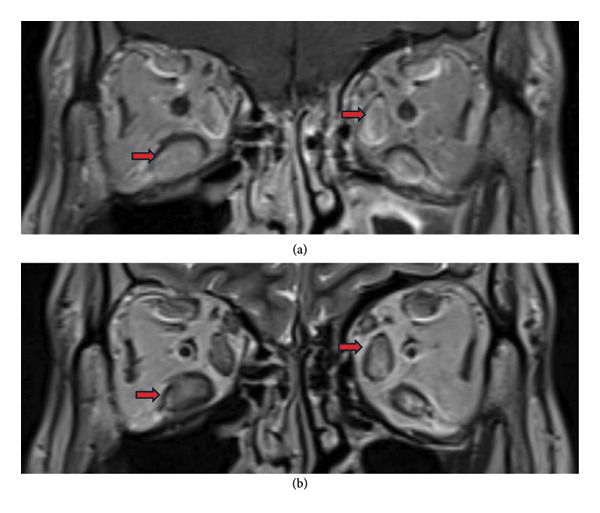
Pre‐ and post‐treatment orbital MRI imaging. Pre‐ and post‐treatment orbital MRI imaging showing extraocular muscle involvement. (a) Representative T2‐weighted coronal MRI images before treatment showing bilateral inferior rectus muscle thickening and enhancement (arrows). (b) Post‐treatment images at 16 weeks showing stable muscle involvement without significant morphological changes. While quantitative differences were not statistically significant due to short follow‐up, the images demonstrate that no progression of muscle fibrosis occurred during treatment. Inner rectus and inferior rectus muscles are the most affected muscles in TED and were marked with red arrows.
